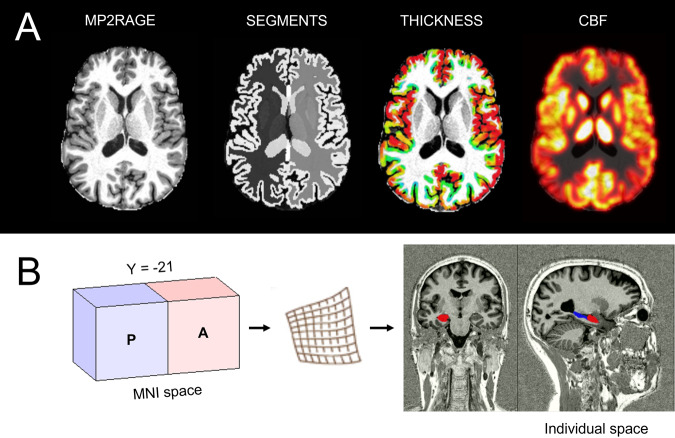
Multimodal MRI measurements. A MRI measurements in volume (segments), cortical thickness, and CBF. B Segmentation of anterior (A) and posterior (B) subparts of the hippocampi.
